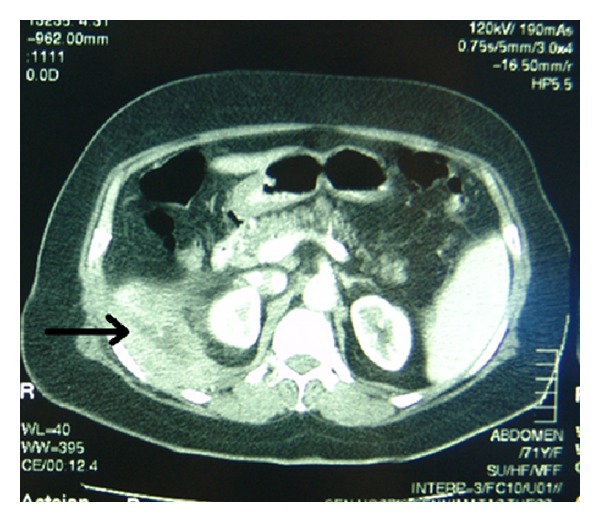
CT scan showing a retroperitoneal abscess (arrow) due to retained gallstones.
